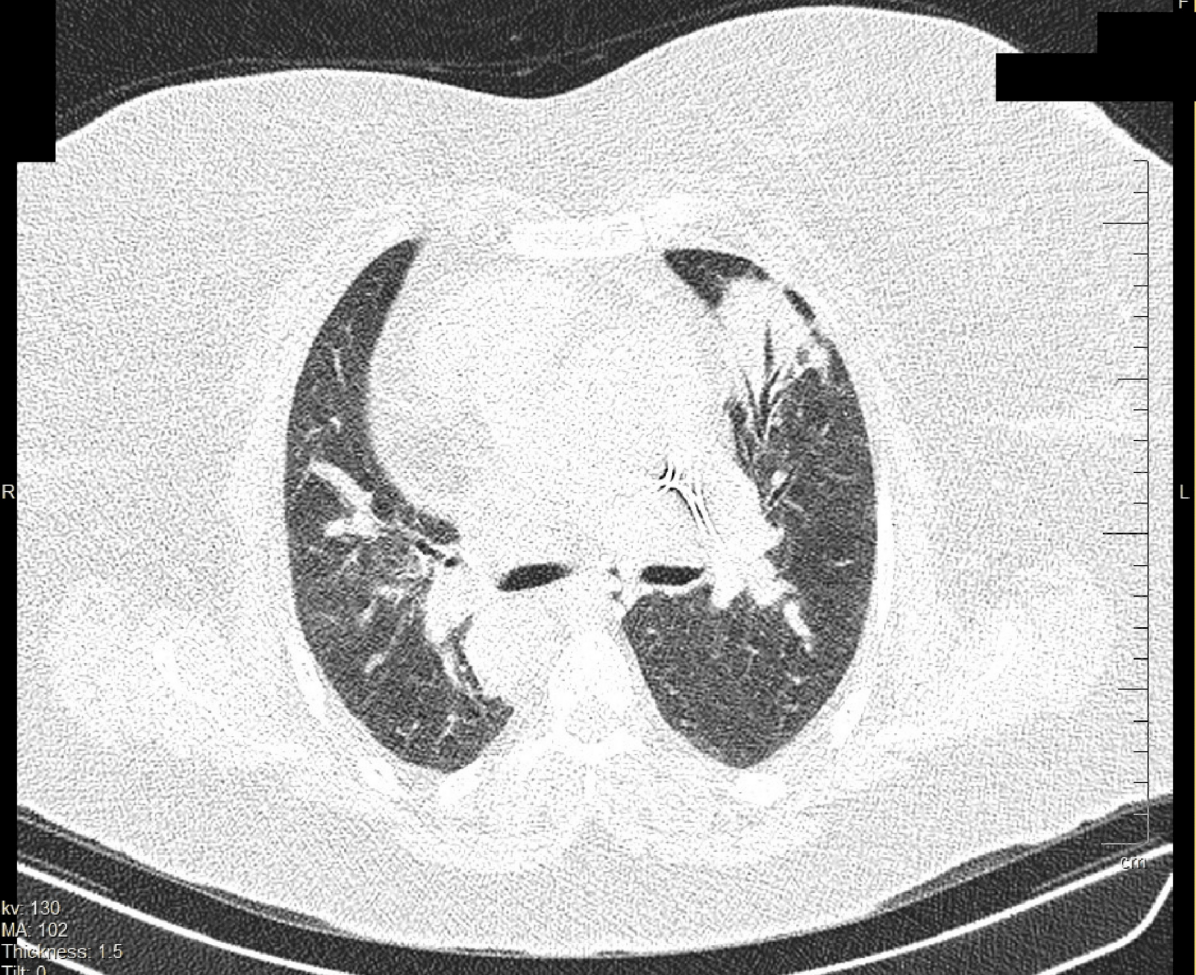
Non-contrast chest CT scan Non-contrast chest CT scan revealed cardiomegaly, septal thickening, and an air bronchogram with a lobar pattern, along with a suspicious mass in the left lung, indicating pulmonary parenchymal involvement. These findings primarily suggest pneumonia and decompensated heart failure as the top differential diagnoses, with pulmonary thromboembolism (PTE) as a secondary consideration.
